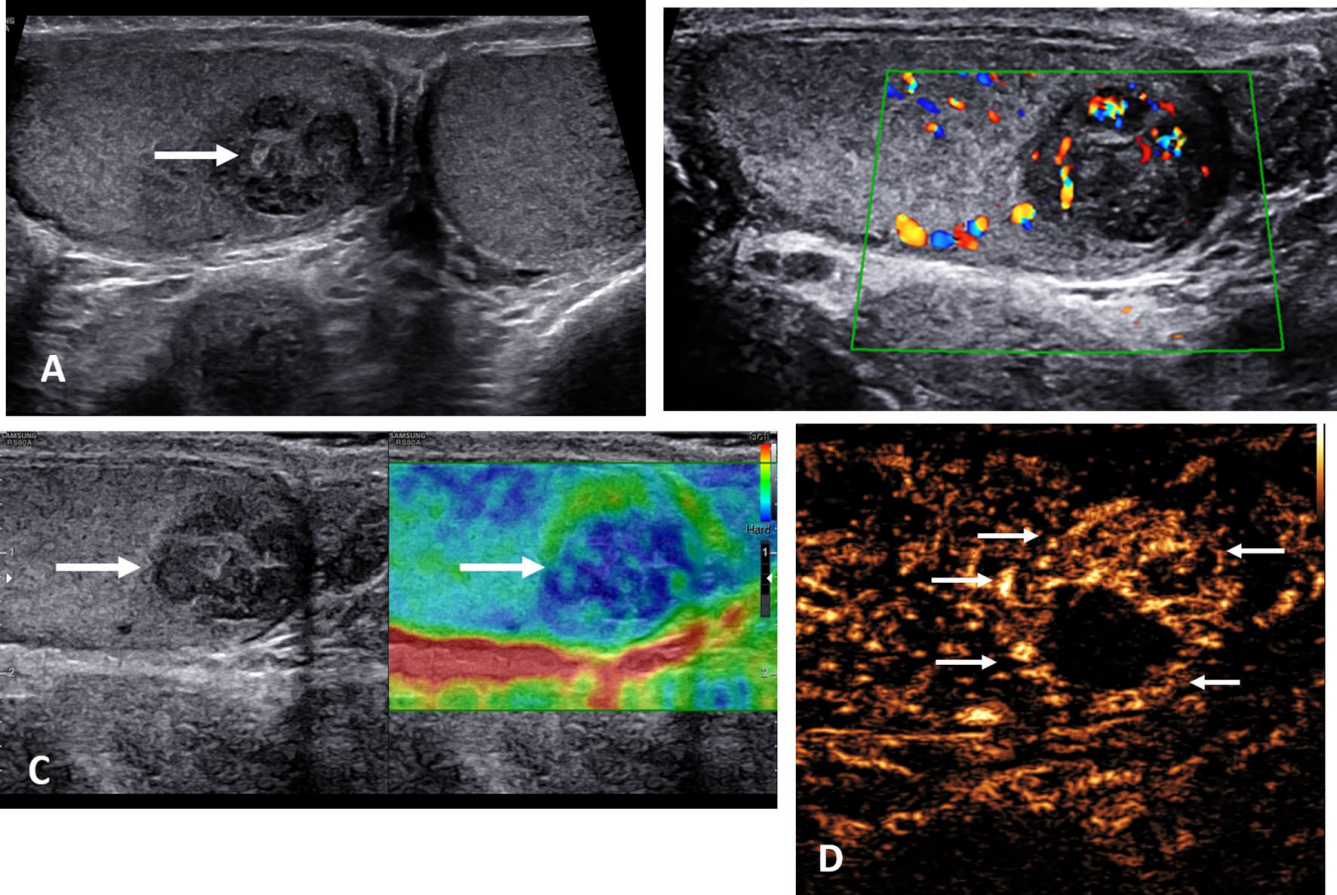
Stage 1 seminoma in a 51-year old. A Transverse view B-mode ultrasound of the testes demonstrating afocal heterogenous lesion in the right testis (arrow). B Longitudinal color Doppler view of the lesion showing intra-lesional color Doppler flow (arrows). C Tissue elastography demonstrates increase stiffness of the lesion, which is predominantly blue (arrows). D A contrast enhanced ultrasound examination demonstrating enhancement of the focal lesion (arrows) with a pocket of avascularity corresponding to an area of tumor necrosis
